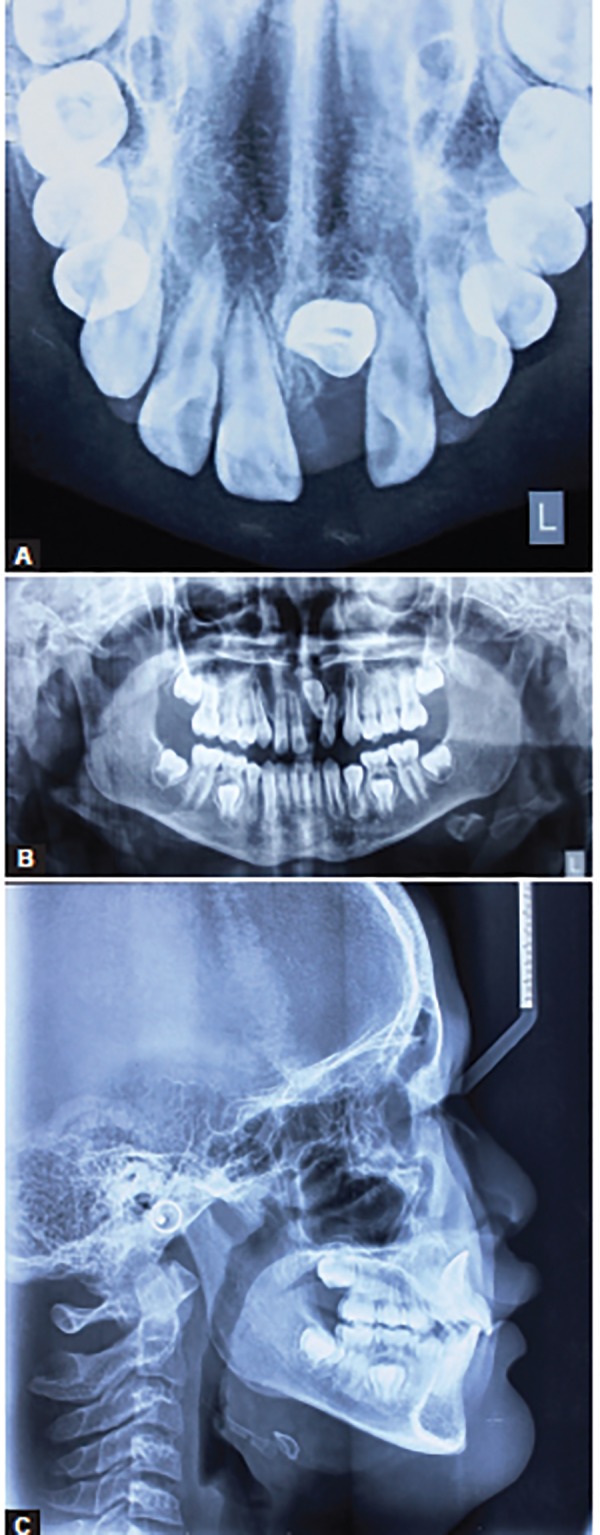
(A) Occlusal view of a patient with maxillary left permanent central incisor dilaceration, (B) panoramic radiographic view of a patient with maxillary left permanent central incisor dilaceration, and (C) lateral cephalogram of a patient with maxillary left permanent central incisor dilaceration
